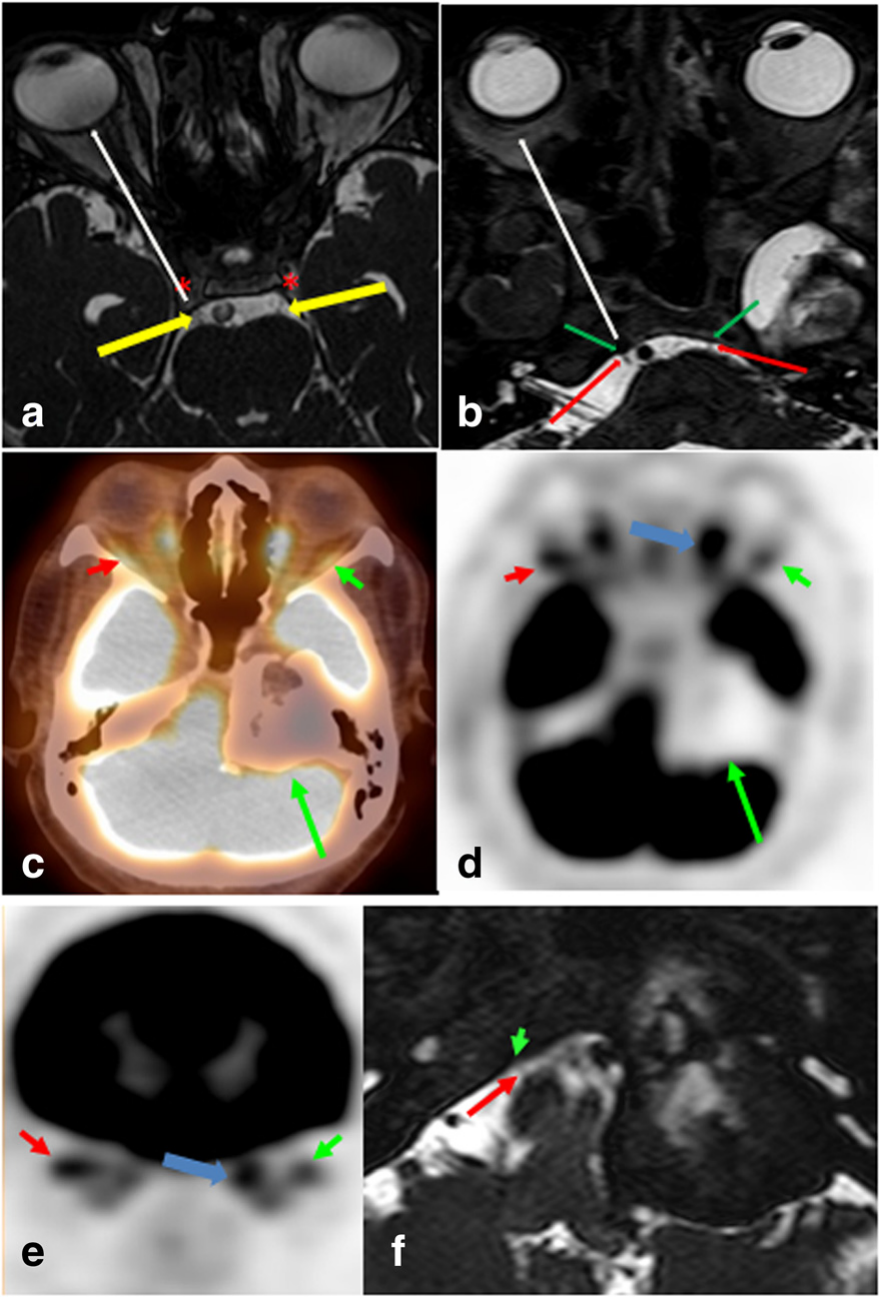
Axial Fast imaging employing steady-state acquisition (FIESTA) MRI image (a) showing the CNIII (yellow arrows) entering the cavernous sinus (asterix). From here, CNIII courses anteriorly in the dura of the cavernous sinus to enter the orbit through the superior orbital fissure (SOF), supplying the extra ocular muscles except the superior oblique and lateral rectus muscles. Axial FIESTA MRI image (b) shows the normal abducens nerves (red arrow) entering Dorello’s canal (green arrow). From here, CN VI courses anteriorly through the cavernous sinus to enter the orbit through the SOF, supplying the lateral rectus muscle. The white arrows show the general direction of travel of CN III and VI (a, b). A 73-year-old male presenting complaining of double vision. Axial fused PET/CT (c), PET (d), and coronal PET (e) images showing a large peripherally ossified hypometabolic expansile mass centered in the left petrous apex extending to the cerebellopontine angle and middle cranial fossa (long green arrow). Note the lower activity in the left lateral rectus muscle (green short arrow) compared to the right one (red arrow) and the overcompensating hypermetabolic left medial rectus muscle, indicative of left abducens nerve paresis which was confirmed clinically. f Zoomed in Axial FIESTA MRI imaging shows the normal right abducens nerve (red arrow) entering Dorello’s canal (green arrow). On the left side, the mass is seen involving the expected location of the left CN VI
